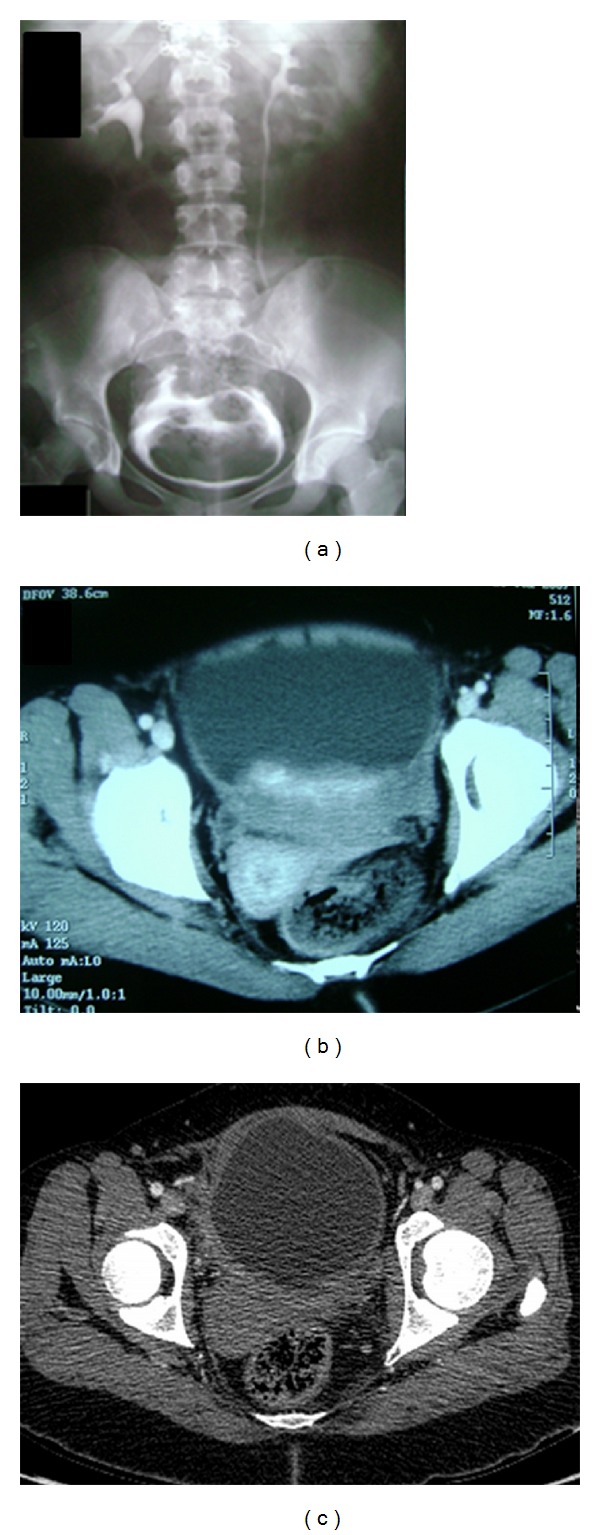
Intravenous pyelography of the patient before operation. A large filling defect can be seen in the bladder (a). A CT image showing a heterogeneous, contrast enhancing mass at the posterior wall of the bladder extending through the ureteral orifices (b). Five years after operation. CT image shows no radiological evidence of the disease (c).
